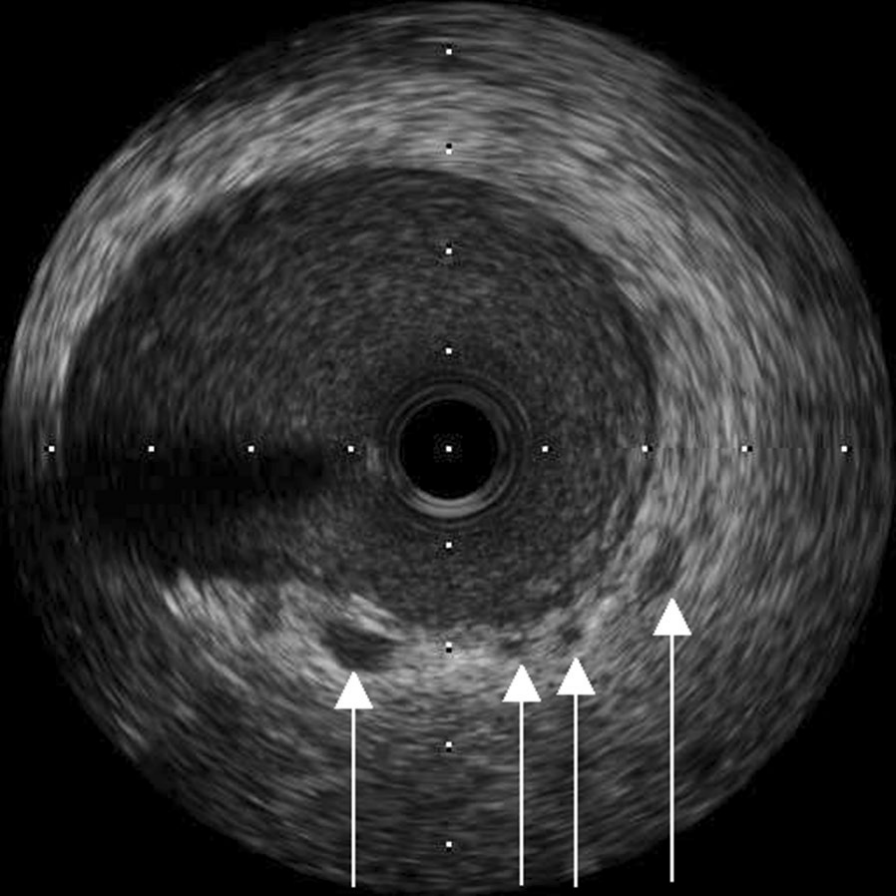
After successful left main stem stenting, intravascular sonography was performed with a coronary guide wire in the left anterior descending artery. This intravascular sonographic view depicts several hypoechogenicities in the vessel wall of the proximal left anterior descending artery (*arrowheads*). In other frames, there was very prominent thickening of the intima in this coronary segment. One example is given as Additional file 2
